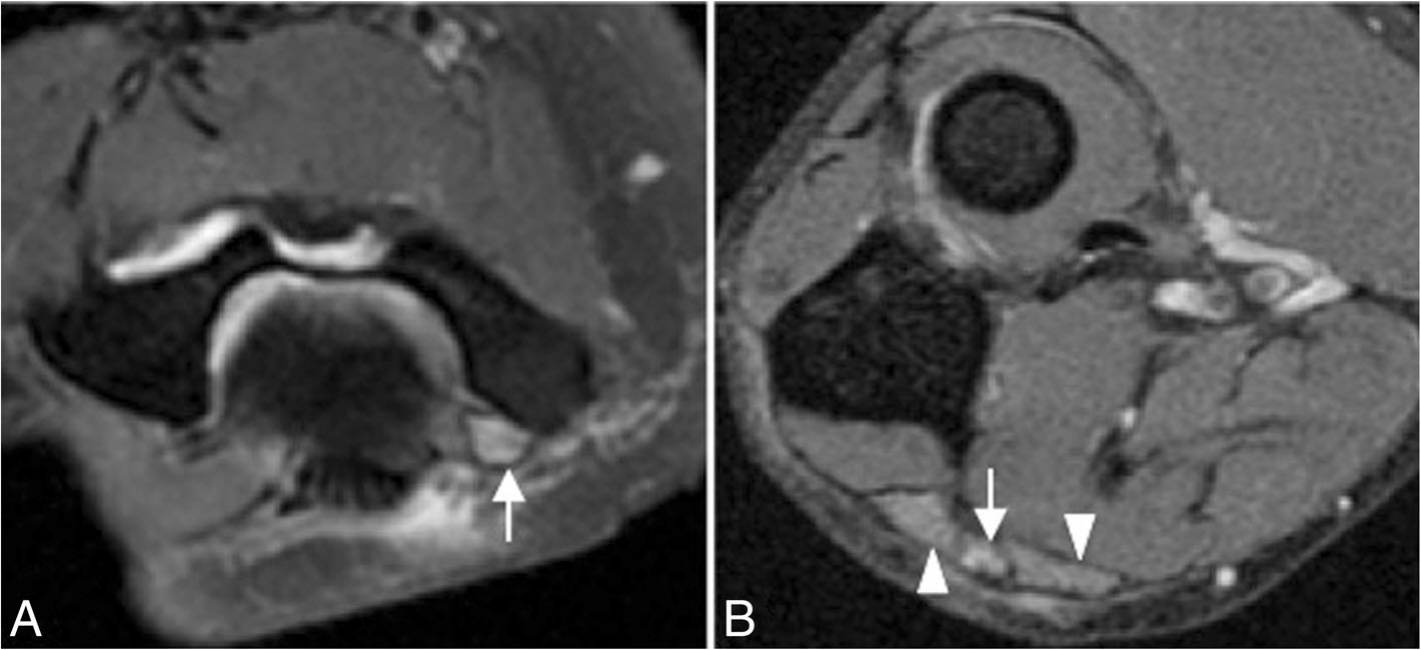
A 60-year-old woman with pain, muscle weakness, and paresthesias. Axial FS PD-weighted MRI (a, b) showing an enlargement and hyperintense ulnar nerve in the cubital tunnel (white arrow) and subacute denervation of the flexor carpi ulnaris muscle (white asterisks)
